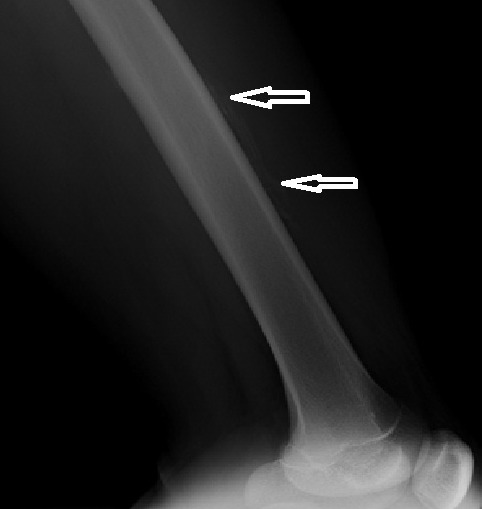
Garçon 16 ans, hémophile B sévère. Radiographie du fémur gauche en incidence de profil montrant des appositions périostées pluri-lamellaires (flèches) médio-diaphysaires
